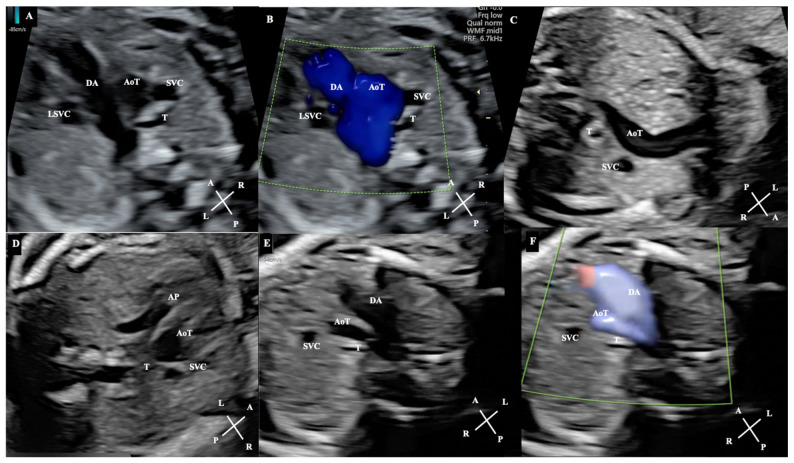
Echocardiographic transverse plane of the fetal thorax at the level of the classic 3VT view. From left (L) to right (R), the ductal arch (*DA*), the transverse aortic arch (AoT) passing to the right of the trachea (T), and the right superior vena cava (SVC) are identified. This view demonstrates a supernumerary vessel on the left side of the Pulmonary Artery (PA) and the *DA*, suggestive of a persistent left superior vena cava (PLSVC). From L to R, the vessels visualized are the LSVC, the *DA*, the AoT, and the right SVC. This configuration represents a “four-vessel” rather than a three-vessel view, shown in a 2D image (A) and with color Doppler (B). In a fetus with Transposition of the Great Arteries (TGA), only two vessels are visualized instead of three. From L to R, these are the AoT and the SVC. The AoT demonstrates rightward convexity as it arises from the anterior right ventricle (RV) (C). In a fetus with Tetralogy of Fallot (TOF), this view shows a smaller pulmonary artery (PA) and an enlarged AoT (D). In a fetus with Aortic Coarctation (CoA), a discrepancy in vessel size is observed, with a larger PA and a smaller AoT shown in a 2D image (E) and with color Doppler (F).
